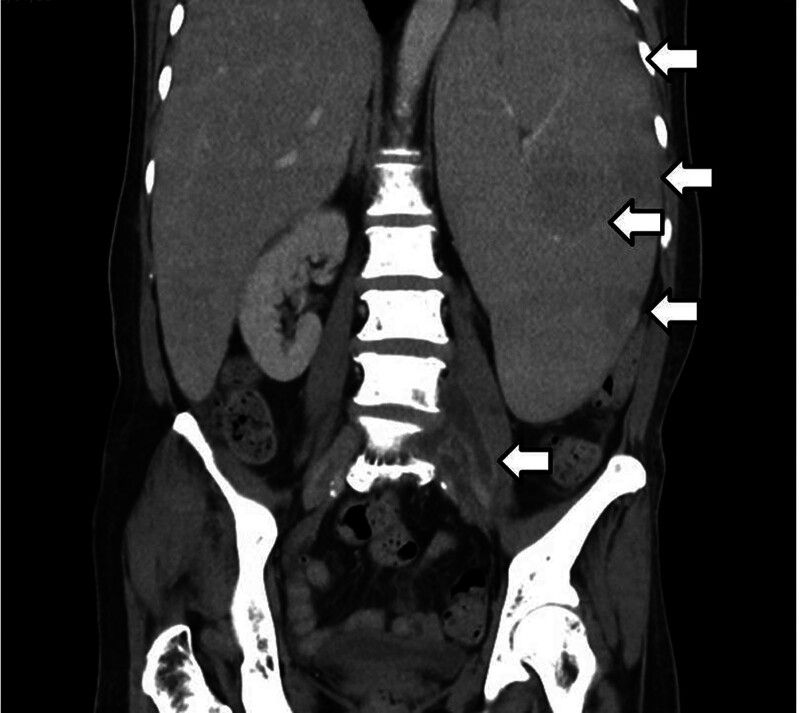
Computed tomography of left psoas abscess and splenomegaly, with multiple poor enhanced nodules. The bony structure revealed mosaic density and compatible with myelofibrosis.
